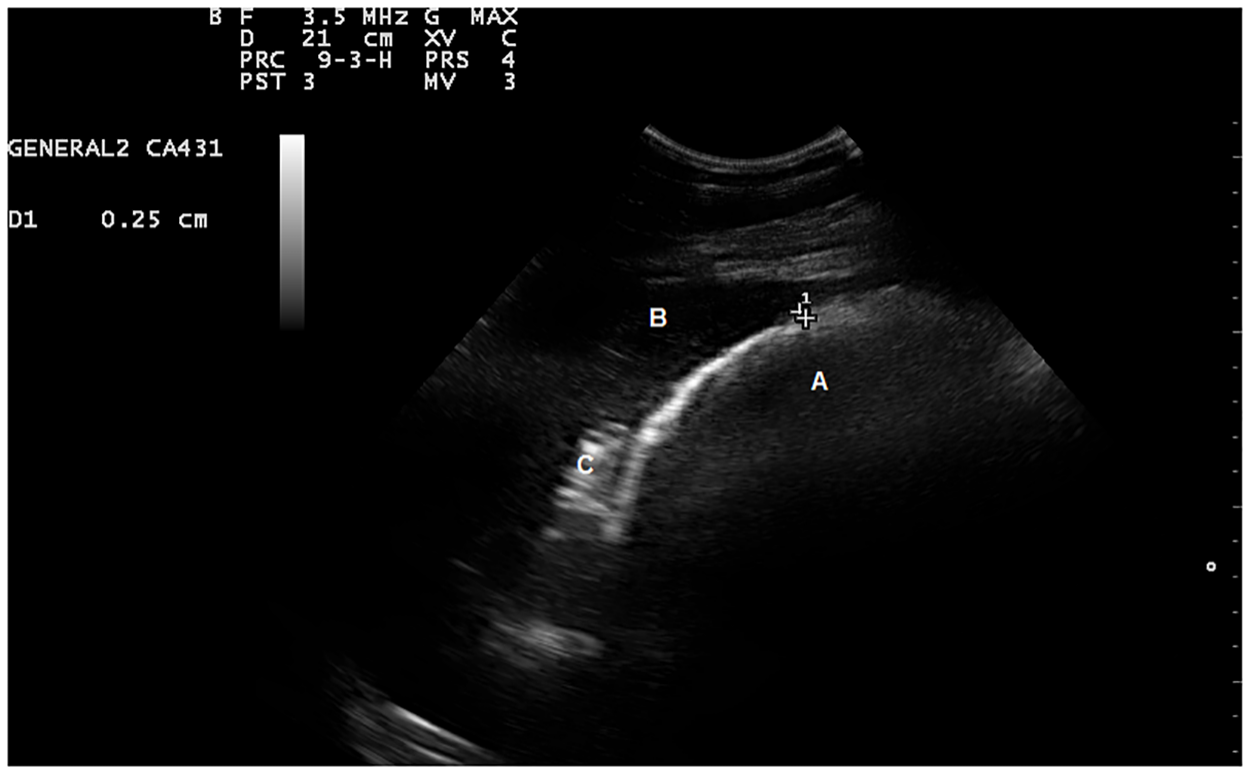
A normal sonogram of the right dorsal colon in a healthy pony. Image obtained by placing a convex transducer in the right 12^th^ intercostal space. The right dorsal colon (A) was identified as a semicircle with bowel gas beam reflections. The liver (B) is seen in the upper hand corner of the image, the duodenum (C) is seen between the liver and the right dorsal colon. The measured thickness of the colonic wall was 0.25 cm.
